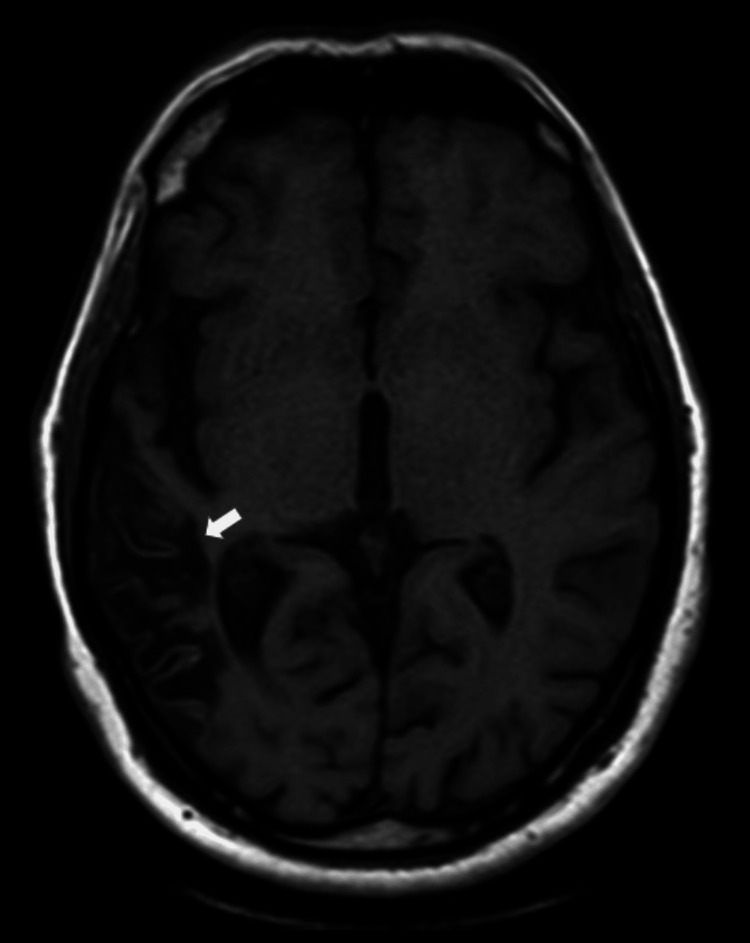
Magnetic resonance imaging (MRI) of the brain T1-weighted image (axial section) showing a hyperintense cortical lesion in the right temporal lobe
